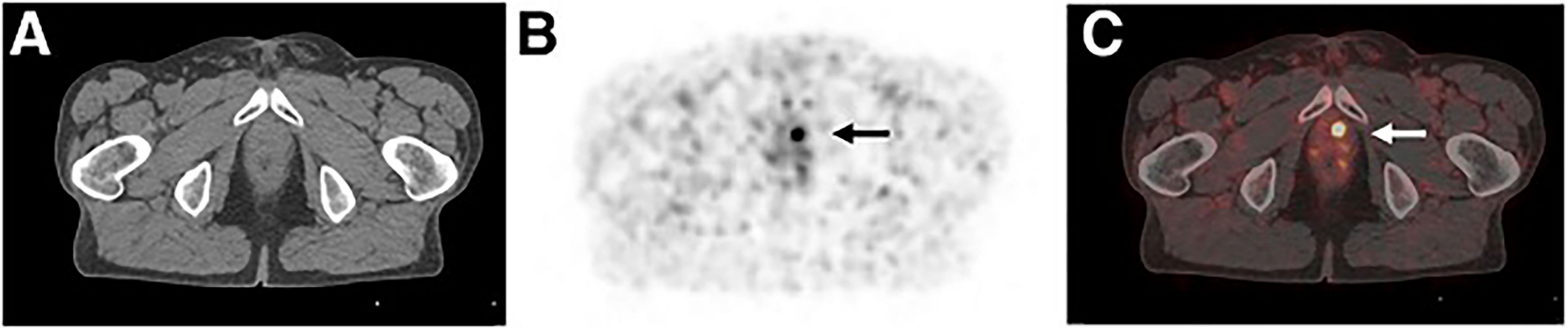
A 78-year-old patient with biochemical recurrence (PSA of 0.54 ng/mL) after radical prostatectomy (initially pT3b N0 M0 R0 G2). ^68^Ga-PSMA ligand PET/CT reveals focal uptake in left paramedian prostatic fossa, indicating local recurrence. The picture below shows transaxial CT (A), PET (B), and fused PET/CT (C) images respectively. Patient was referred for salvage radiation treatment. This research was originally published in JNM. Author(Schwarzenboeck SM, Rauscher I, Bluemel C, Fendler WP, Rowe SP, Pomper MG, Afshar-Oromieh A, Herrmann K, Eiber M). PSMA Ligands for PET Imaging of Prostate Cancer. J Nucl Med. 2017 Oct;58(10):1545-1552.^©^ SNMMI.
